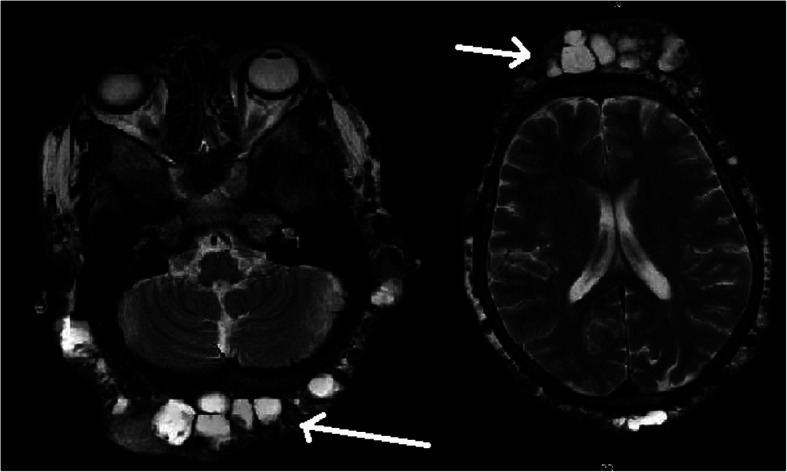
T2-weighted axial MRI images of the brain showing multiple confluent and discrete solid cystic lesions of trichilemmal tumor of the scalp (arrows)
